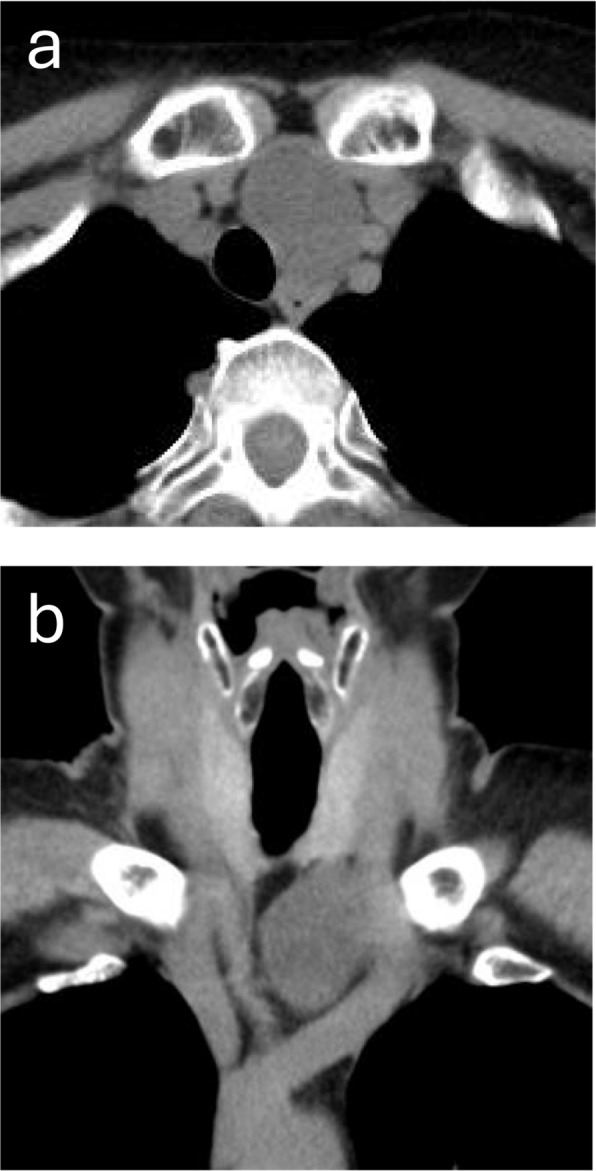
Contrast-enhanced computed tomography revealed a well-circumscribed, 4-cm mass located in the left paratracheal region (a, axial view). The lesion displaced and compressed the trachea medially without evidence of invasion into adjacent structures (b, coronal view)
